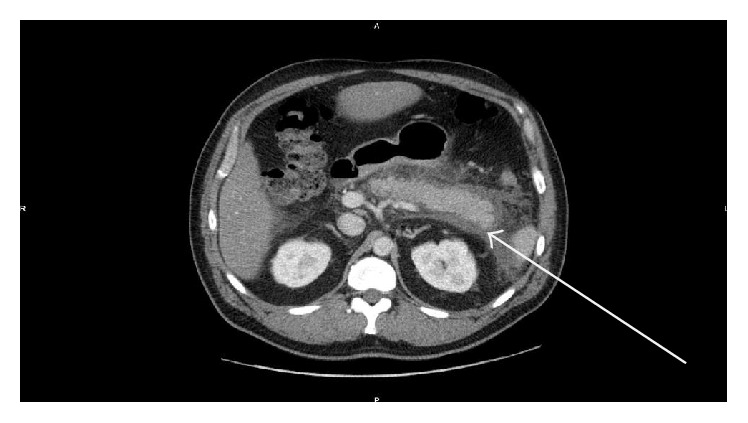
Axial contrast CT scan of the abdomen showing retroperitoneal and peritoneal effusion arising from the tail and the body of the pancreas 4 days after admission.
